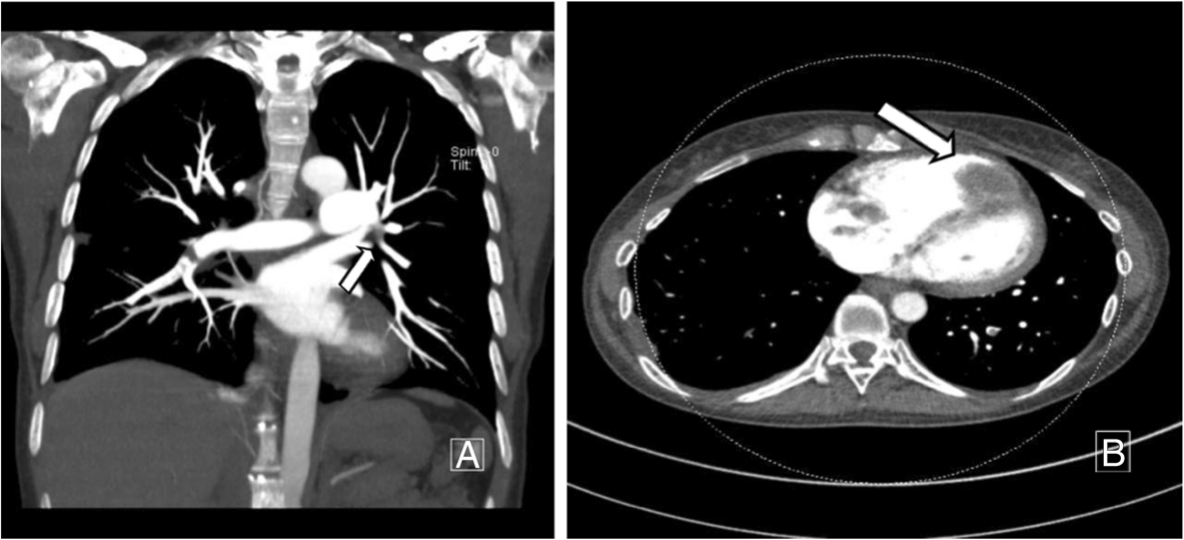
Deep vein thrombosis three-dimensional CT (DVT 3D CT) angiography showing the cardiac mass and thromboemboli before the surgery. (A) Maximum intensity projection (MIP) image shows pulmonary artery thromboembolism involving the right lower lobar artery and its segmental branches. (B) Computed tomography (CT) angiography of the pulmonary artery shows multiple large thrombi in the right atrium and right ventricle.
